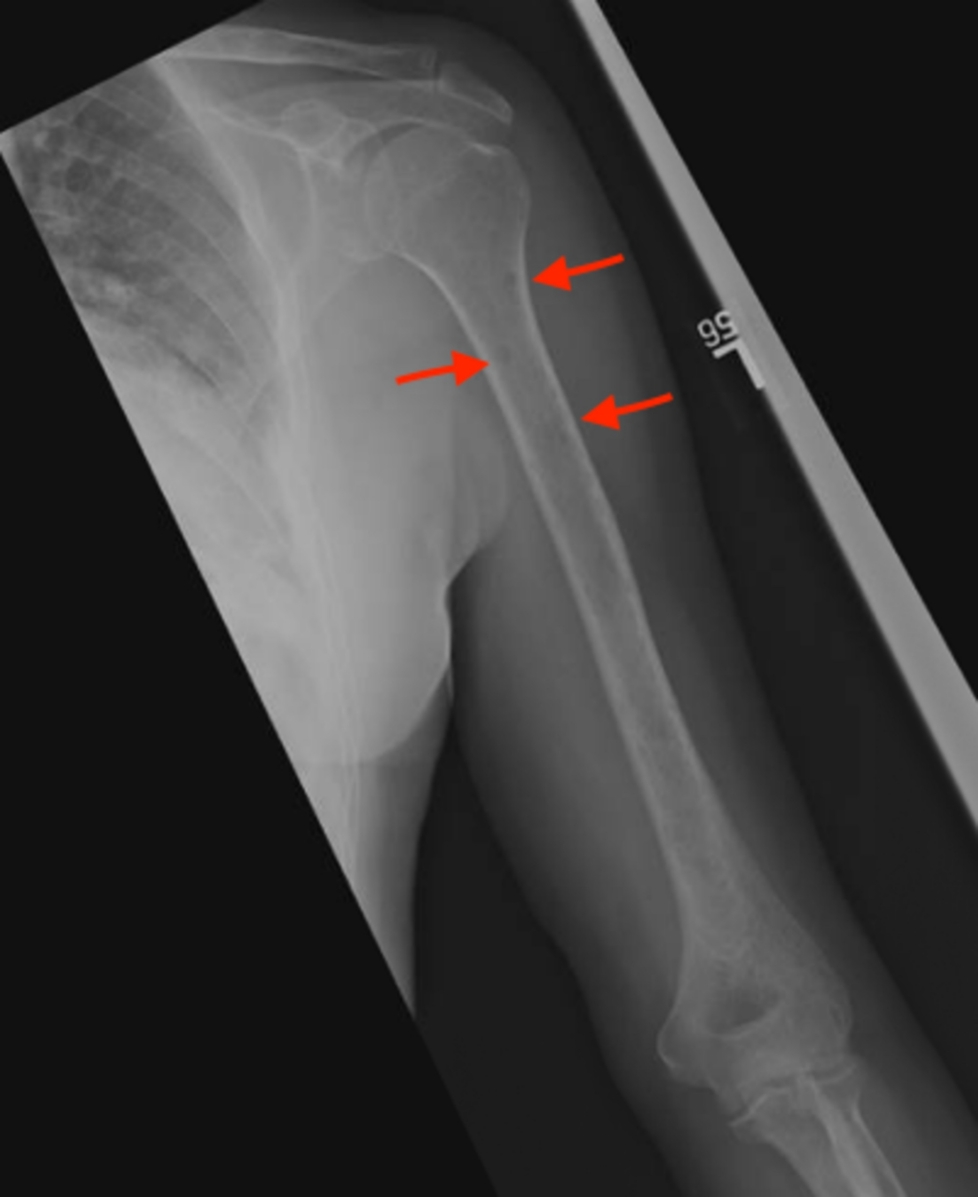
X-ray of the left humerus revealing multiple round, lytic lesions (red arrows), suggestive of multiple myeloma
